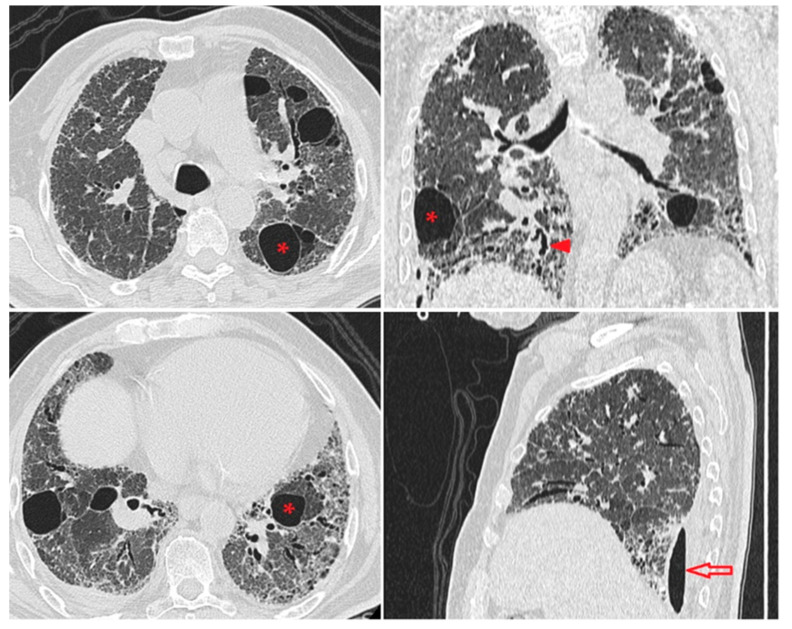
Chest high-resolution computed tomography (HRCT): diffuse “ground-glass”; subpleural reticular opacities associated with traction bronchiectasis (red arrowhead); multiple large aeric cysts in both lungs (*); loculated right lung pneumothorax (red arrow). A 70-year-old male was referred to our expert center for chronic dry cough and dyspnea on exertion (climbing stairs and walking short distances) that has slowly progressed over the last two years. He was a non-smoker with no relevant occupational exposures. His medical history revealed a previous right hydropneumothorax that was drained a year ago in a thoracic surgery department. He had multiple cardiovascular comorbidities (arterial hypertension, chronic ischemic cardiopathy, stented right coronary artery for a previous anteroseptal myocardial infarction) and type 2 diabetes treated with oral antidiabetics. The physical examination showed an overweight patient (BMI = 28.4 kg/m^2^), pallor with cyanosis at minimal exercise, grade 3 finger clubbing, lower extremity edema, basal bilateral Velcro crackles, and right pleural friction. He had oxygen saturation (SpO_2_) of 88% on room air, which increased to 92% with oxygen supplementation. Routine hematology and biochemistry investigations were unremarkable. Echocardiography revealed concentric left ventricular hypertrophy, dilation of the right ventricular cavity, stage II tricuspid regurgitation, and pulmonary hypertension (non-invasive estimation of pulmonary artery systolic pressure was 45 mmHg). Lung function tests showed a severe restrictive pattern with best forced vital capacity (FVC) of 42% pred., best forced expiratory volume in one second (FEV1) of 47% pred., and Tiffeneau–Pinelli index (FEV1/FVC) of 0.82. The chest X-ray showed reduced lung volumes with diffuse ground-glass opacification and multiple bilateral airspace opacities. Additional evaluation by chest HRCT revealed extensive bilateral fibrotic changes and cystic damage of the pulmonary parenchyma, suggestive of chronic interstitial lung disease (ILD). Clinical features, pulmonary function tests, and chest CT evaluation disclosed a longstanding undiagnosed ILD with a cystic appearance. Due to age, poor cardiologic status, and comorbidities of the patient, surgical procedures such as decortication with the re-expand of the lung and sampling of the pulmonary parenchyma could not be performed.
